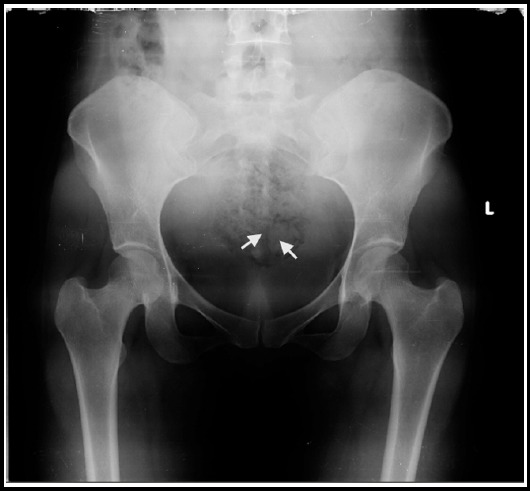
X-Ray pelvis showing radiopaque shadows.
